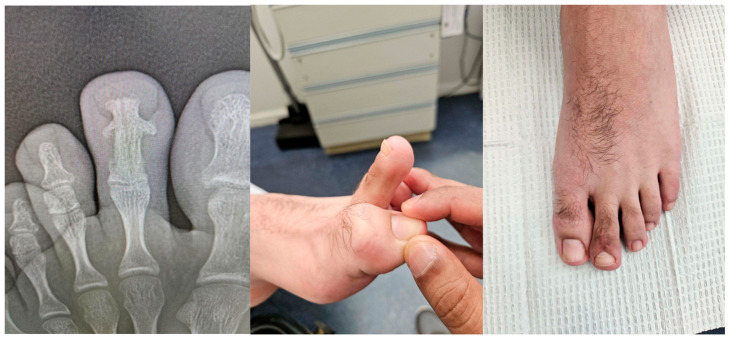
Three-month postoperative radiograph demonstrating solid fusion at the distal interphalangeal joint.
